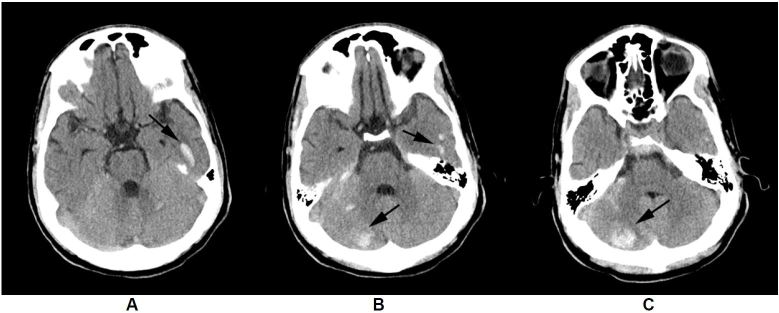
Non contrast CT head demonstrating left temporal and right cerebellar hemorrhages.
